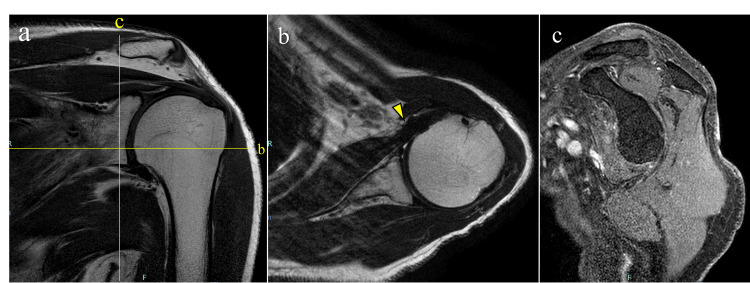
Magnetic resonance imaging at one year postoperatively. (a) Coronal T2-weighted image demonstrating no evidence of residual or recurrent tumor in the axillary pouch. (b) Axial T2-weighted image showing no tumor in the deep layer of the subscapularis muscle; the repaired subscapularis muscle is continuous (arrowhead). (c) Sagittal contrast-enhanced fat-suppressed T1-weighted image demonstrating no abnormal contrast enhancement suggestive of tumor recurrence.
